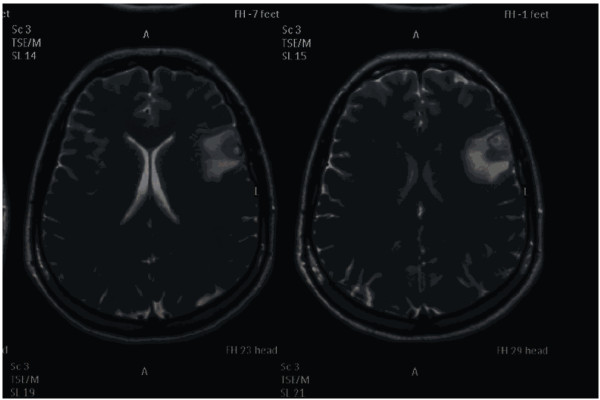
MRI of the brain of the patient in case 2 showing a single cystic enhanced lesion.
